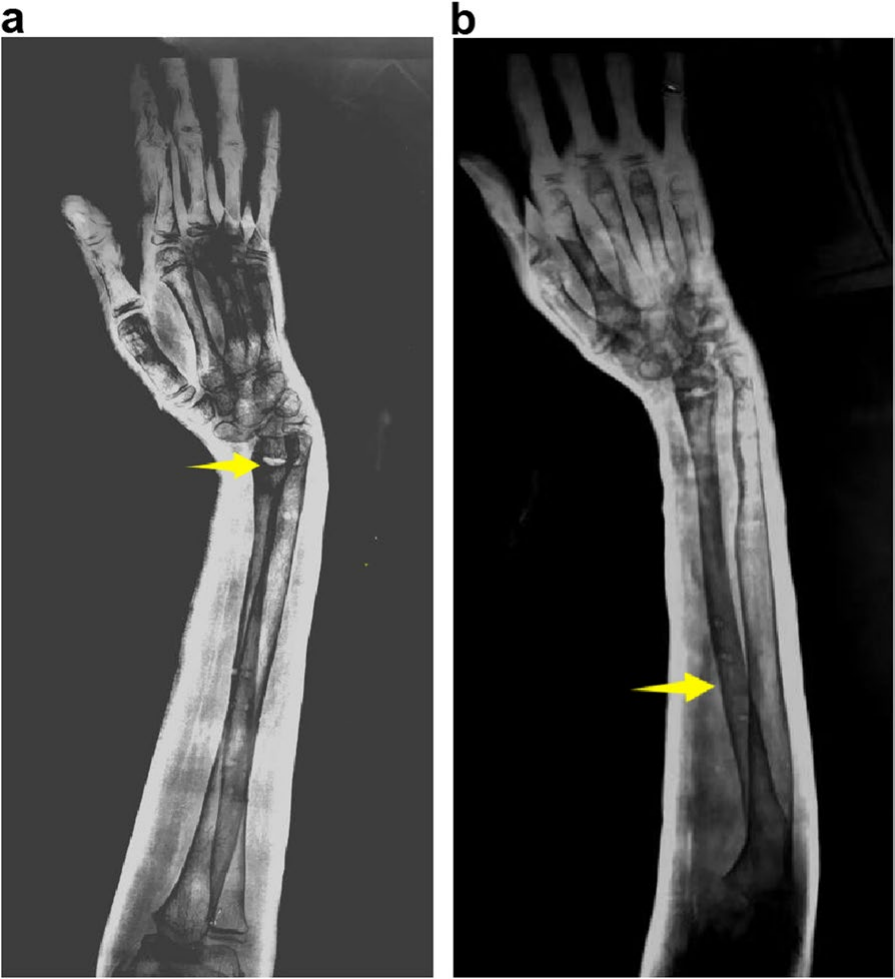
a Radiographs showing the growth plate after 12 months (yellow arrow) and b bone union (yellow arrow), with radial volar subluxation of the wrist (a, b)
